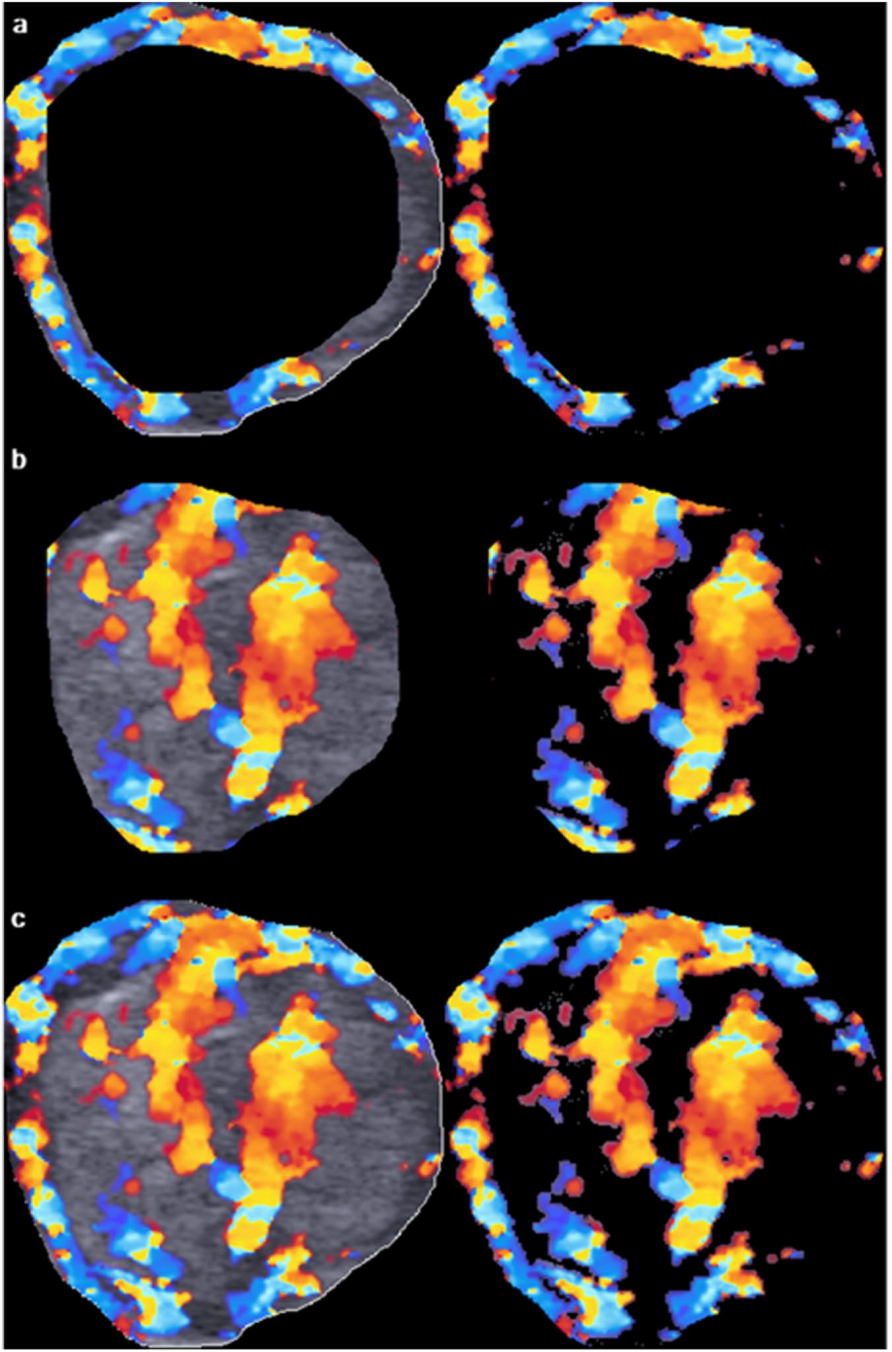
Image analysis of peripheral, central and overall vascular index (VI) of the thyroid nodule as shown in Fig. 2. The primary region of interest (ROI), i.e. the thyroid nodule, was extracted from the outlined area. Using an offset level of 10%, the peripheral (a, left) and central (b, left) regions of the nodule were segmented and the total number of pixel within the segmented area was counted by the computer algorithm. The color pixels coded by the color Doppler ultrasound were extracted by eliminating the grey scale pixels, and the color pixels were counted by the algorithm (a, right and b, right). The VI of peripheral and central regions of the nodule was the percentage of the number of color pixels to the total number of pixels within the segmented area. The overall VI of the nodule was evaluated by counting the total number of pixel within the ROI (c, left) and the number of color pixel in the image with the grey scale pixels eliminated (c, right).
